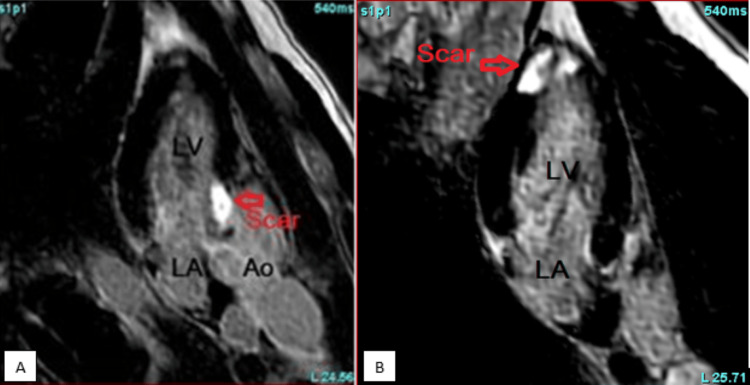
Cardiac magnetic resonance imaging showing transmural late gadolinium enhancement in the basal septum (A) and significant late gadolinium enhancement in the apical inferior and apical lateral region (B).
